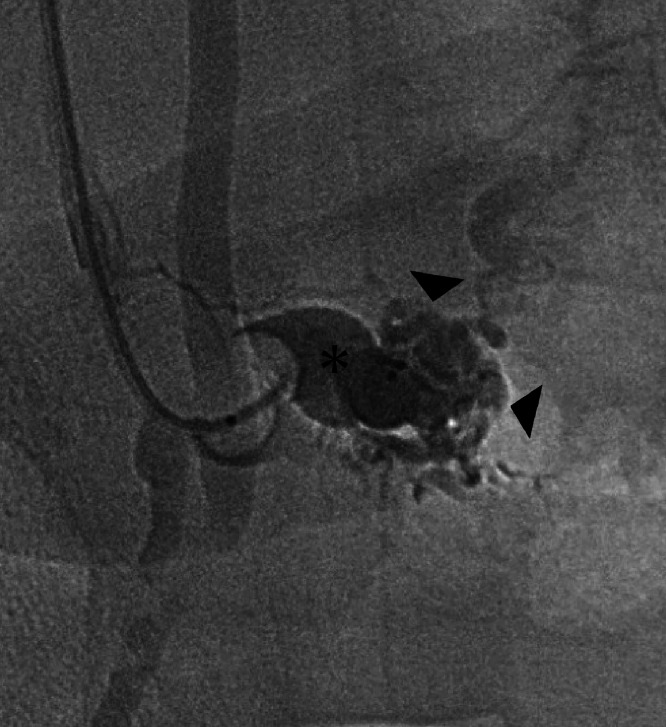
Digital radiography image with balloon occlusion of the dominant outflow vein, a blood drainage channel, from a percutaneous transhepatic route, and retrograde contrast. Multiple nidi (black arrowheads) via the dominant outflow vein (asterisk) are confirmed, leading to the diagnosis of an arteriovenous malformation of Type II in the Cho classification.
